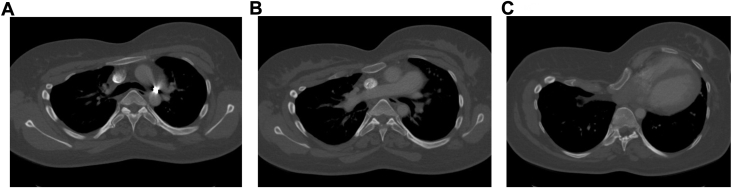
Contrast-enhanced computed tomography scan of the chest with moderate asymmetrical pectus excavatum and platythorax type chest. (A) Axial image of the sternum at the level of the carina. (B) Axial image at the level of the truncus of the pulmonary artery showing a rotation of the sternum. (C) Axial image at the deepest point of the pectus excavatum at the level of the ventricles.
